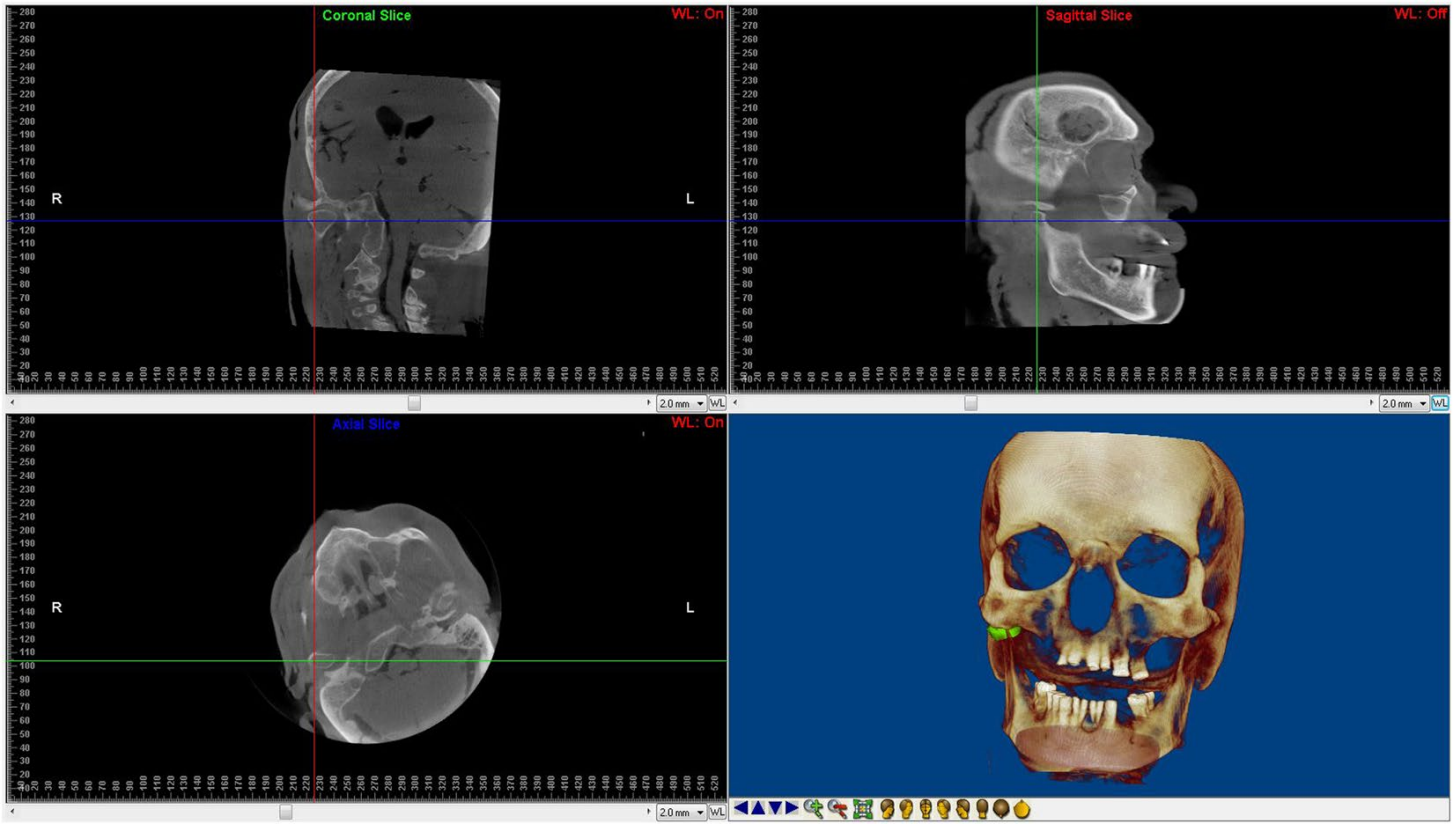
CBCT image orientation and slice selection for linear measurement calculations.
